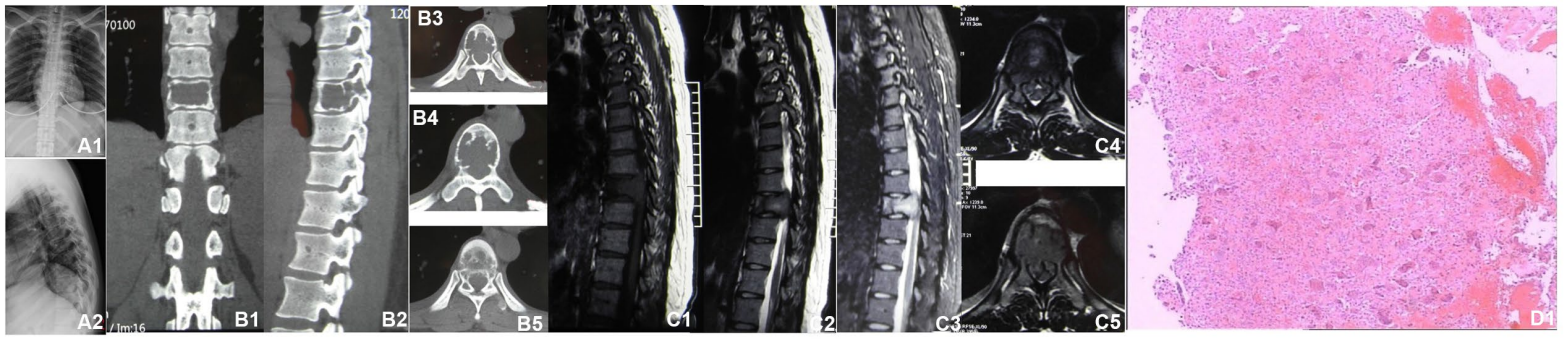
Imaging data at the initial clinic visit (2020-04). (A1,A2) Thoracic vertebral X-ray: The local density of the T9 vertebrae was decreased. (B1–B5) Thoracic vertebral CT: T9 vertebral bone destruction, cortical destruction at the upper, lower, and posterior margins, bilateral bone destruction of the pedicle, an irregular soft tissue density shadow visible in the vertebral body, and the spinal canal were occupied, with a corresponding spinal canal sagittal diameter narrowing. (C1–C5) Thoracic vertebra MRI: T9 vertebral body flattening, and long T1 and long T2 signals appearing within the vertebral body. The fat suppression phase revealed a high signal intensity, bilateral pedicle involvement, bone destruction at the posterior edge of the vertebral body, soft tissue space, and dural compression. (D1) Puncture pathology revealed massive osteoclasts, considering the giant cell tumor of the bone.
